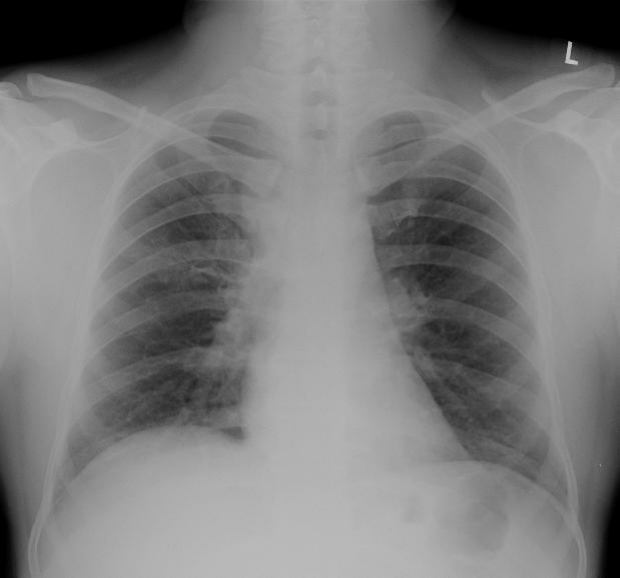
Chest X-ray showing widening of the right paratracheal stripe.
